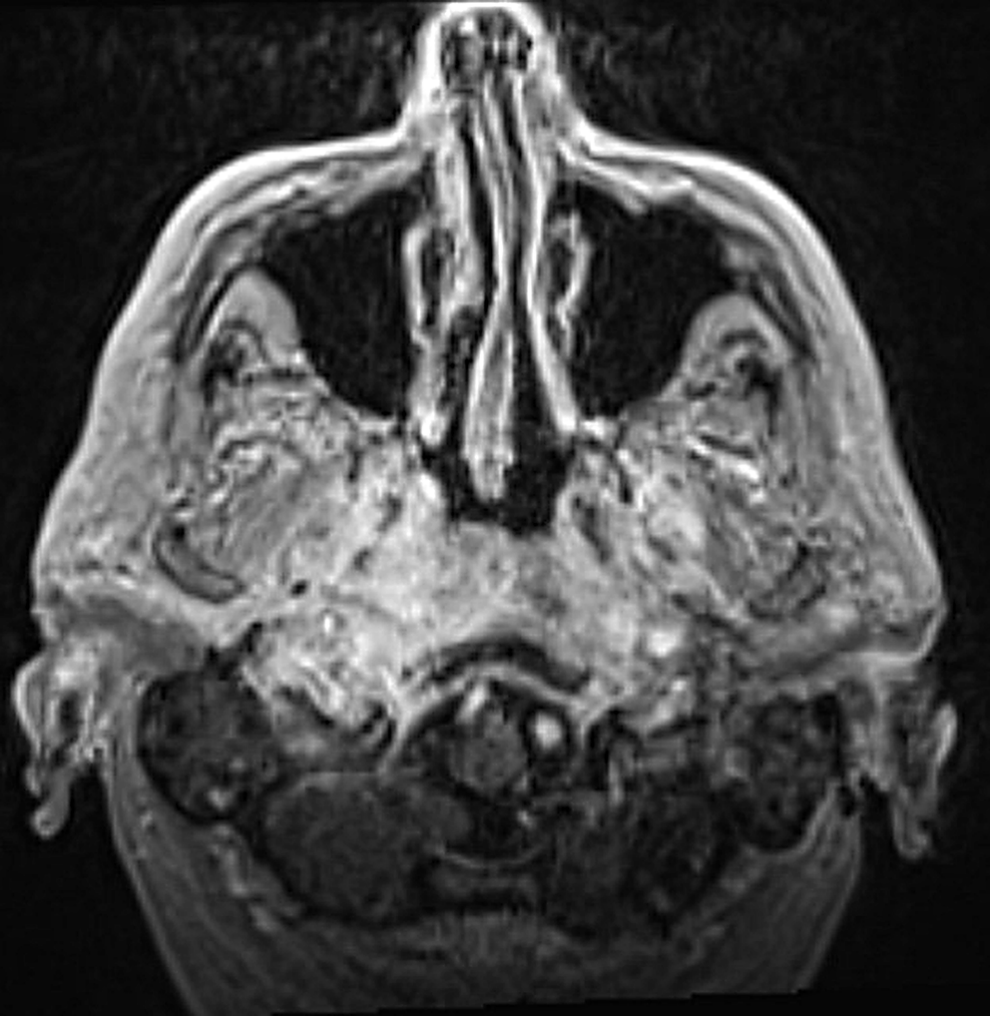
MRI examination after 6 months (ASBO)
